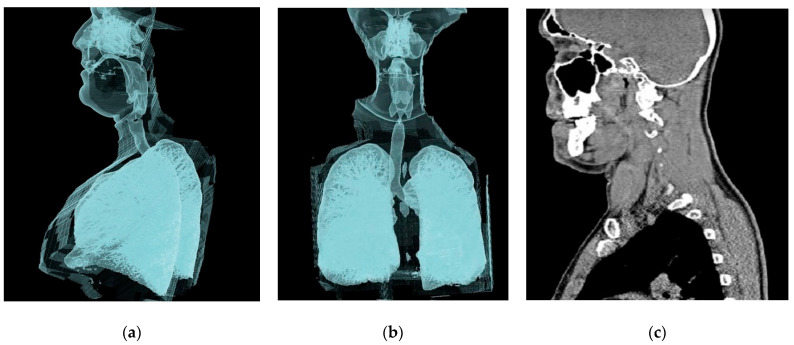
Different views of the computed tomography (CT) images used in this study: (a) 3D render, sagittal view; (b) 3D render, coronal view; (c) CT image scan, sagittal view.
